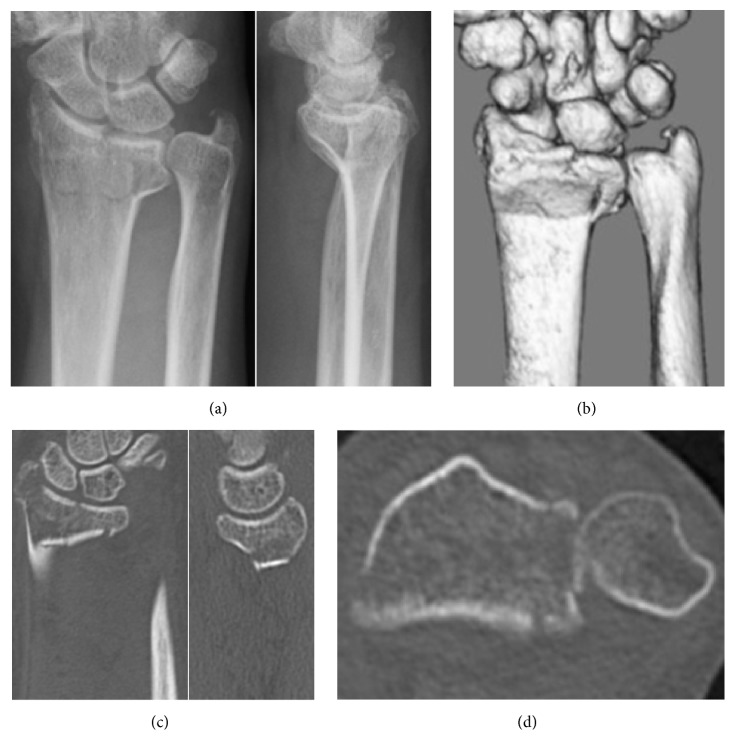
Patient 2. Plain radiographs (a) and CT (b, c, d) at the time of injury. A 12 × 12 mm VLF fragment is observed, with volar displacement.
